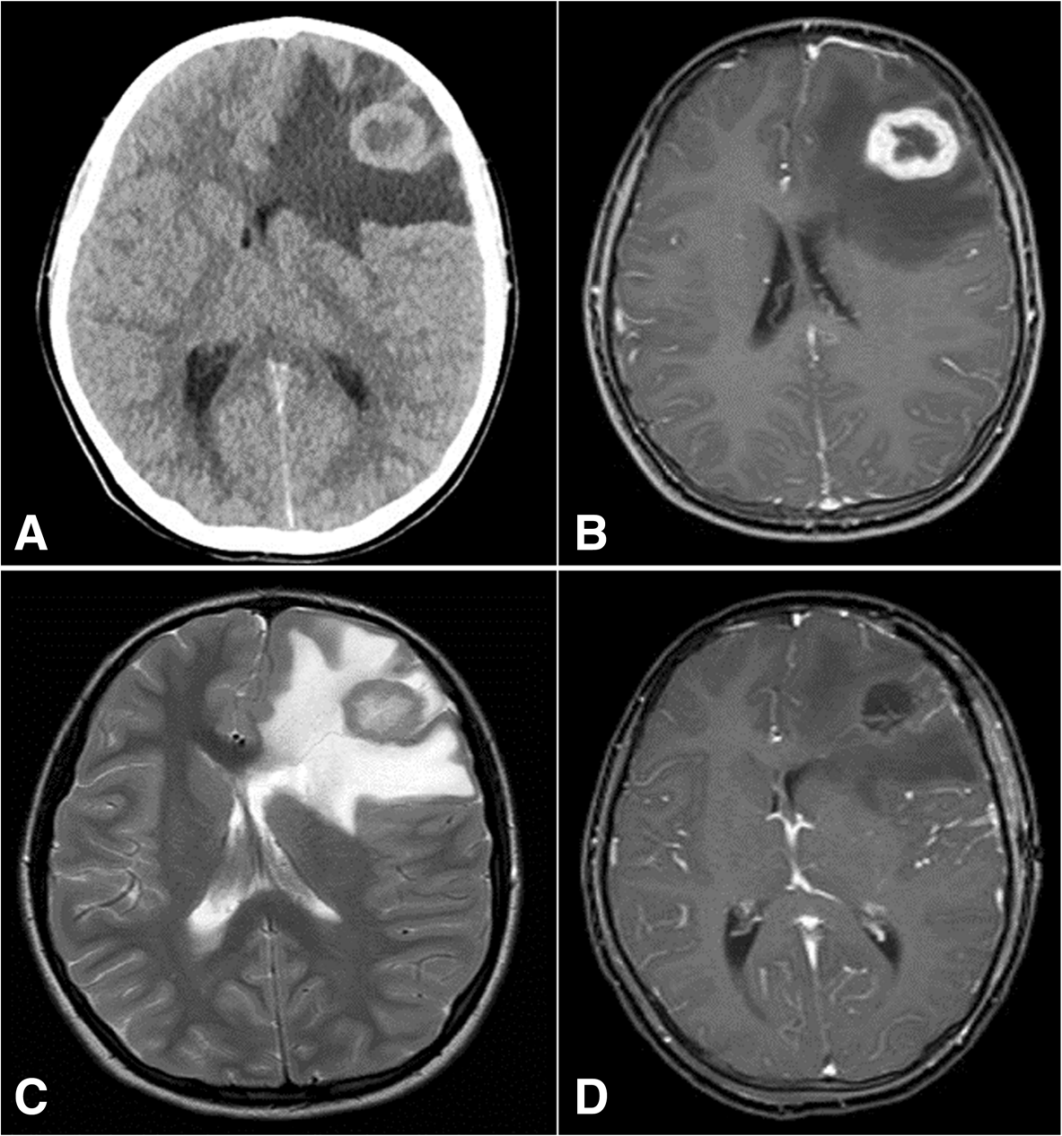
Brain metastasis of lung adenocarcinoma at diagnosis on non-enhanced computed tomography scan (a), post-contrast T1-weighted (b), fluid-attenuated inversion recovery magnetic resonance imaging (c) and after surgical resection on post-contrast T1-weighted (d)
